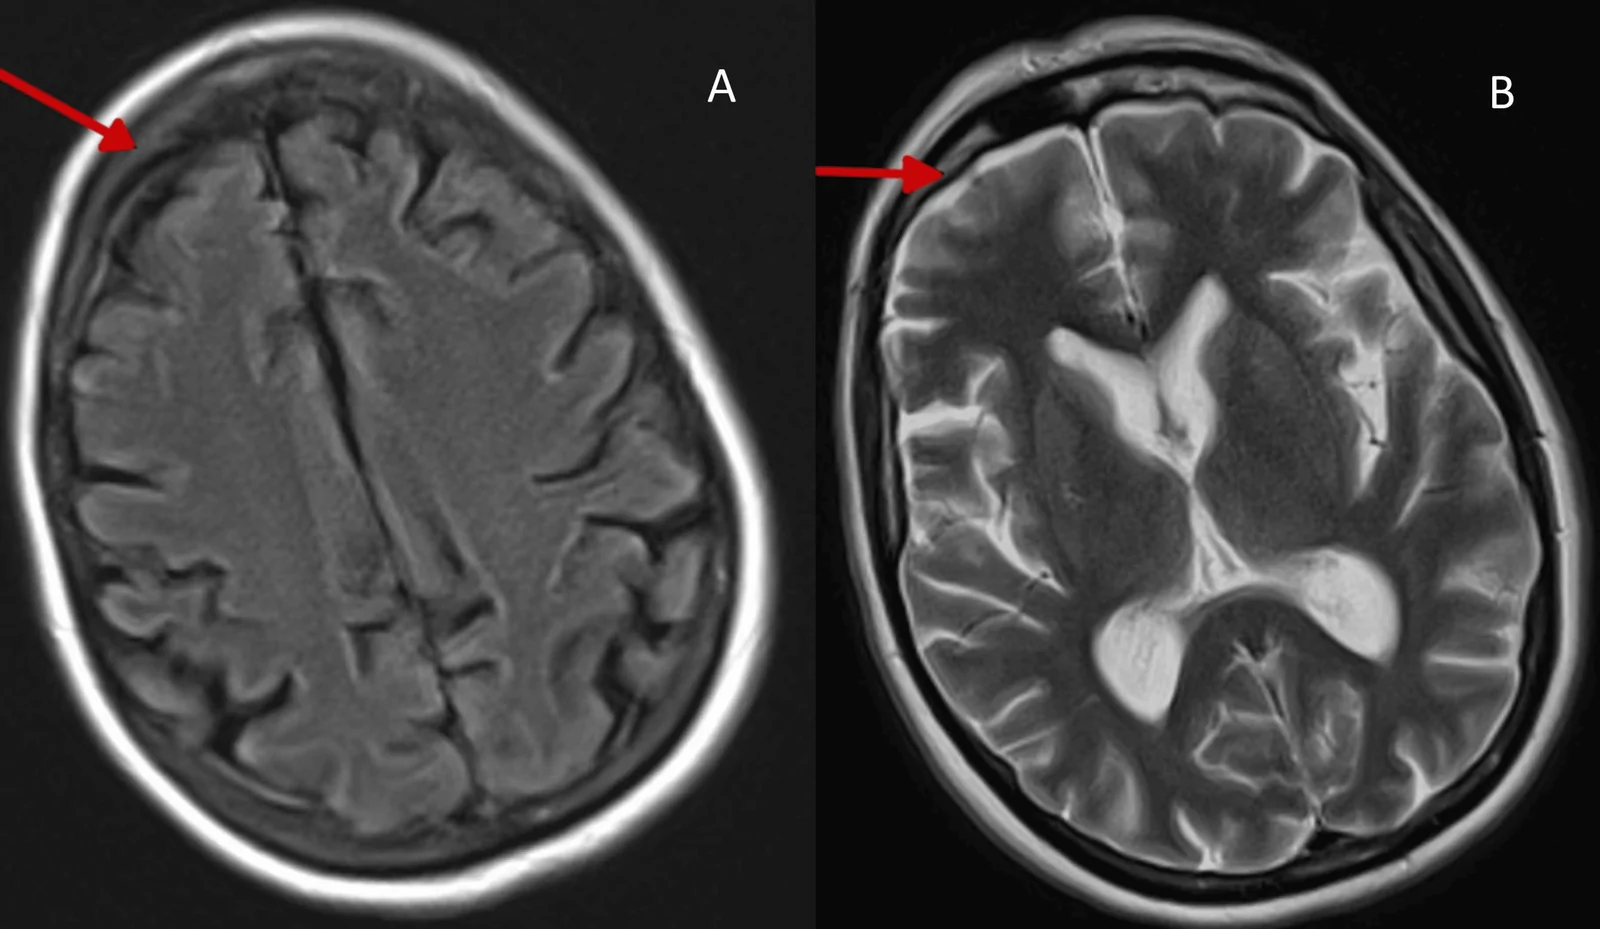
CT Head (A) and MRI Brain (B) A: No acute features. There is significant generalised volume loss for a patient of this age. B: No acute abnormal findings. Generalised parenchymal volume loss is indeterminate for the cause.
